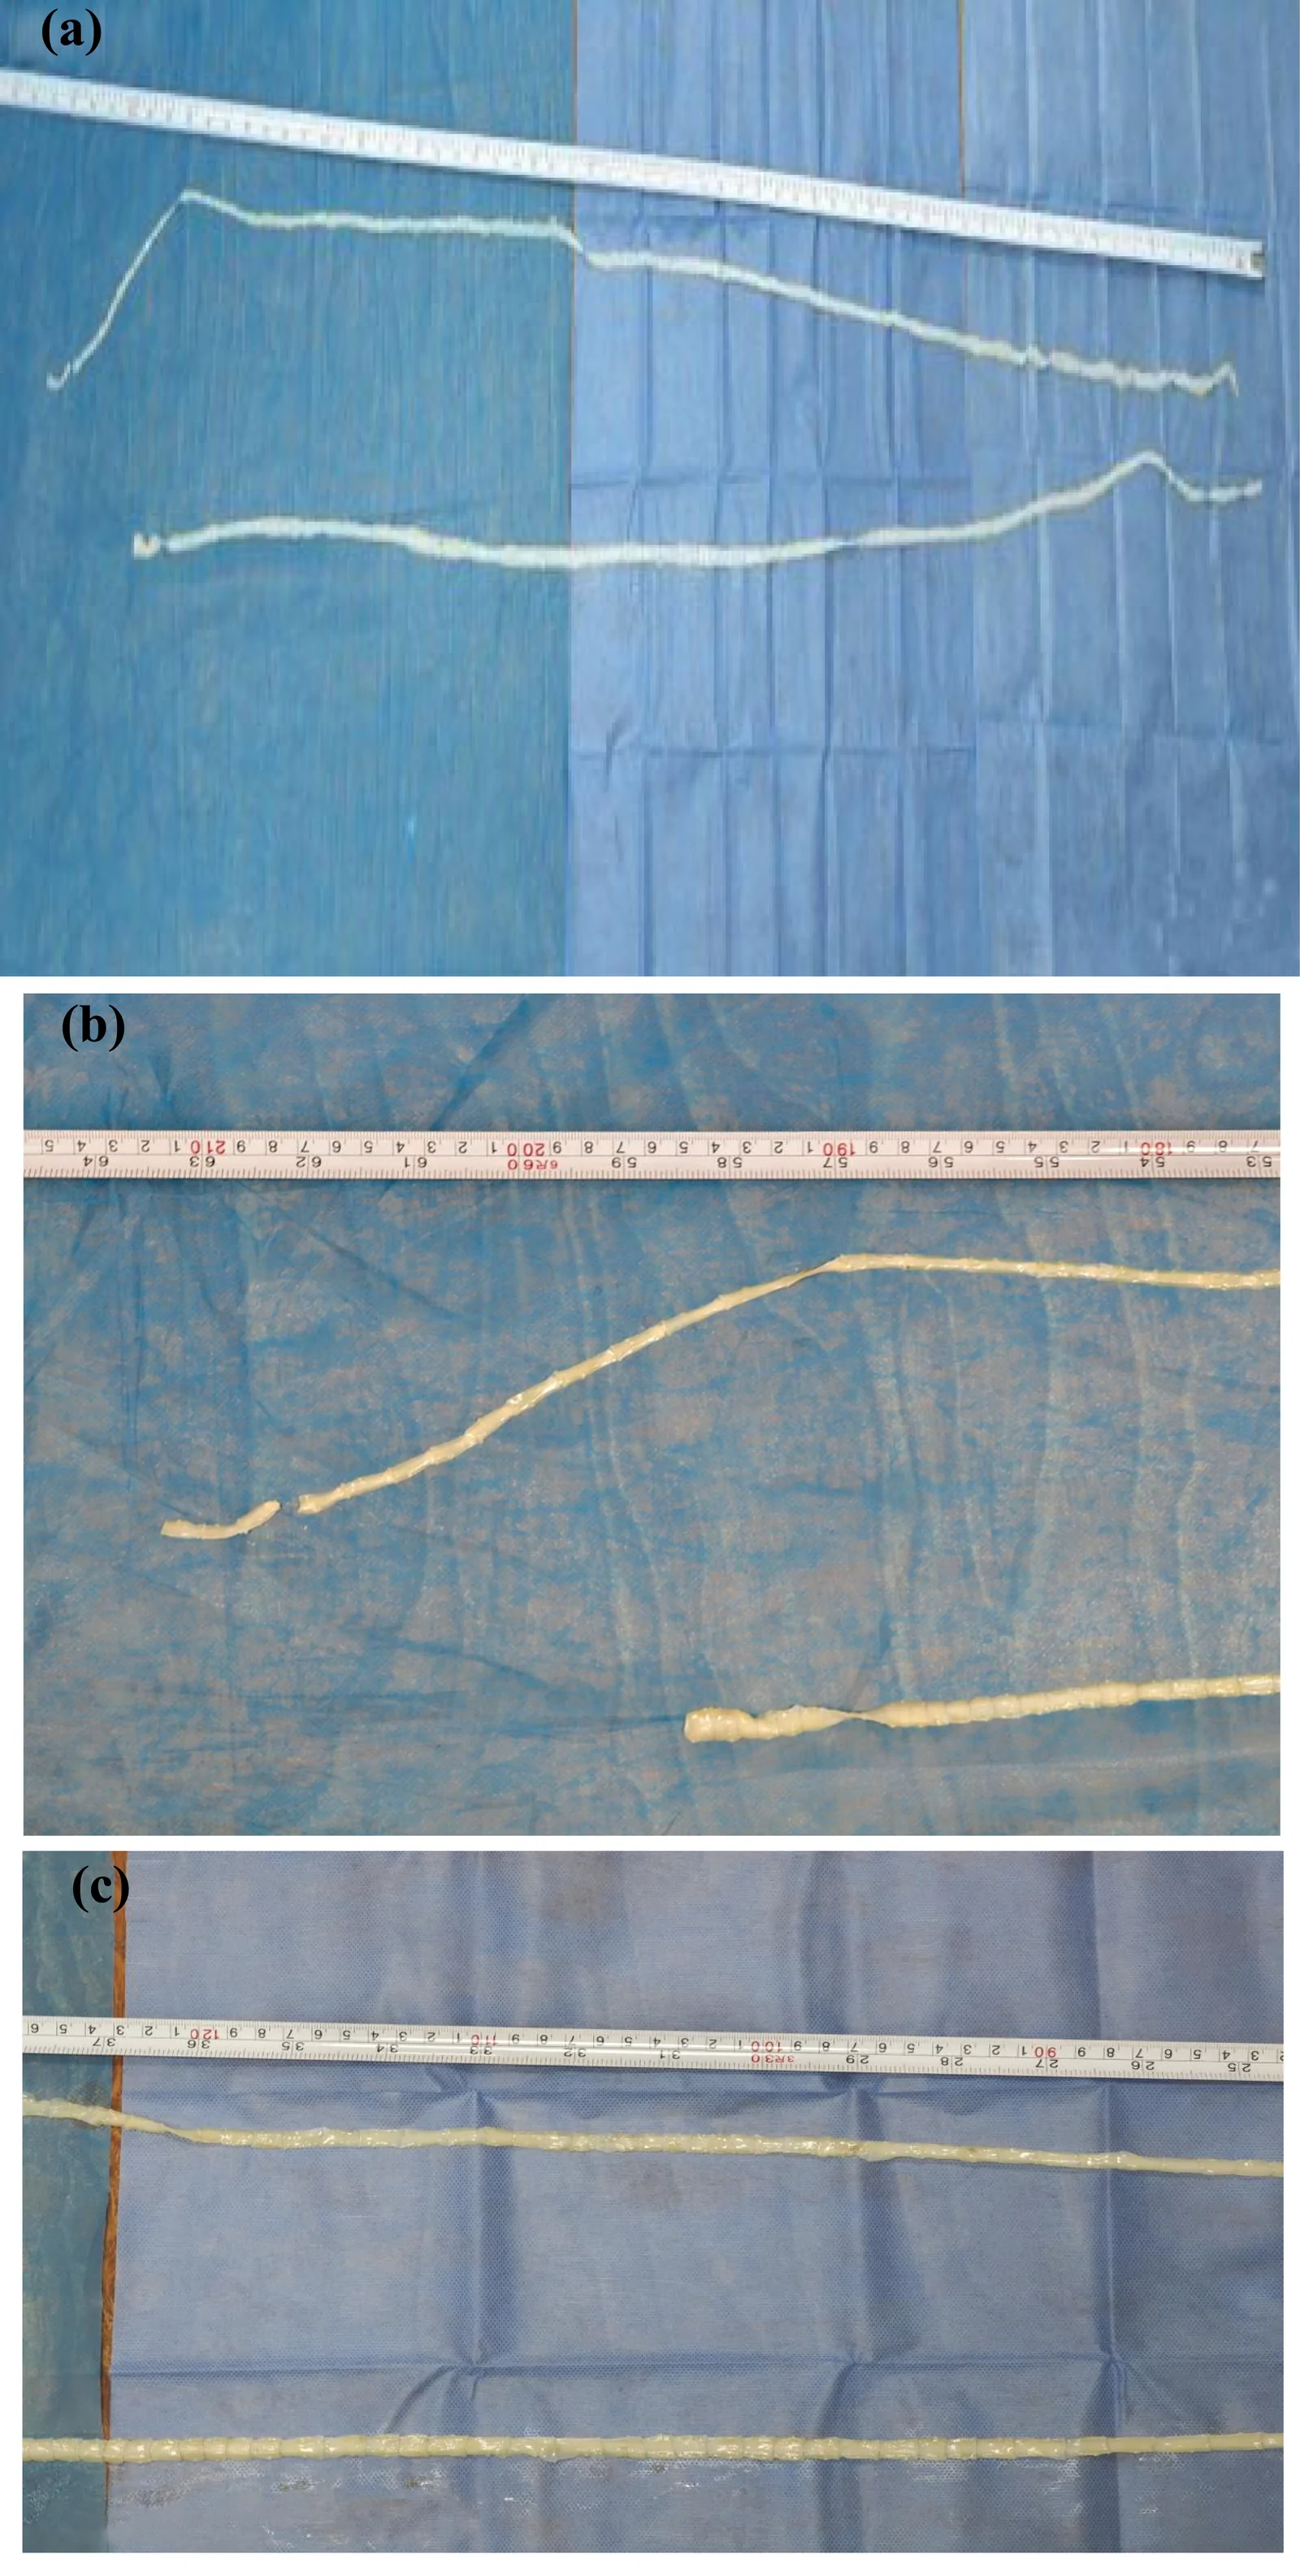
After the CT examination, the patient vomited two white worms.
